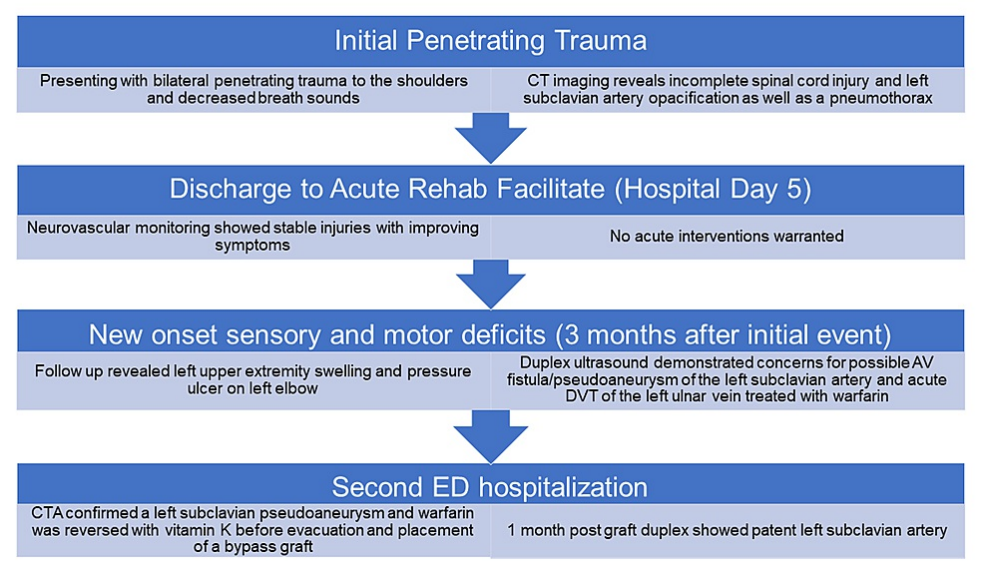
Patient History. CT, computed tomography; LOS, length of stay; AV, arteriovenous; DVT, deep vein thrombosis; CTA, computed tomography angiography
